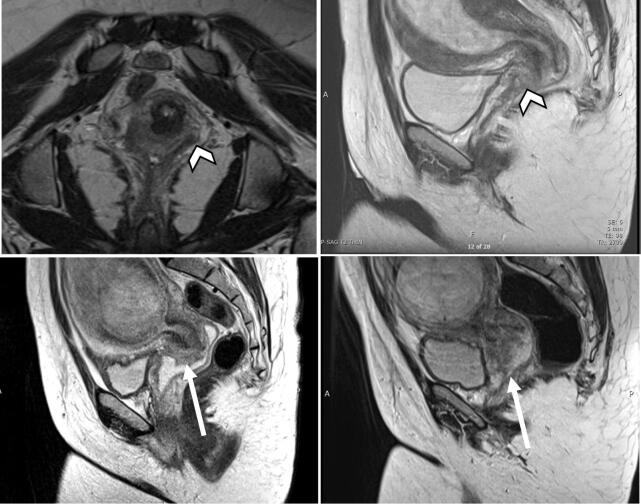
2018 FIGO stage IB1 disease progression to IB3 disease. (a, b) Axial oblique (a) and sagittal (b) T2-weighted image depicts (arrowhead) an exophytic cervical mass involving the anterior lip measuring 1.6 × 1.8 × 1 cm. (b) Sagittal T2-weighted image reveals (arrow) enlarging exophytic cervical mass measuring 1.8 × 3 × 1 cm. (c) Sagittal T2-weight image depicts (arrow) enlarging exophytic cervical mass of the anterior lip measuring 3.2 × 4.2 × 1.8 cm.
